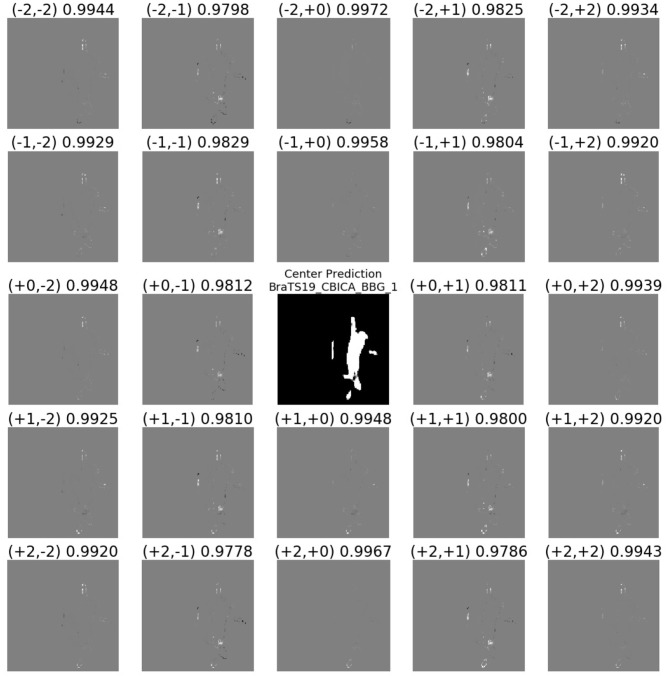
Demonstrating the translational variance of the 2D BraTS model. The center shows the prediction of the model on a 128 × 128 center crop of the MRI. Note the entire tumor fits within this tile. The surrounding subplots show the difference in the prediction as the MRI input crop is offset by one or two pixels in either dimension of the slice. The differences between the predictions in the overlapping pixels show that even the smallest translation in the input can create a difference in the output. Gray pixels indicate no difference in the predictions of the overlapping pixels between the center crop and the offset crop, black pixels are in the offset-crop prediction but are not present in the center-crop prediction, and white pixels are in the center-crop prediction but are not present in the offset-crop prediction.
